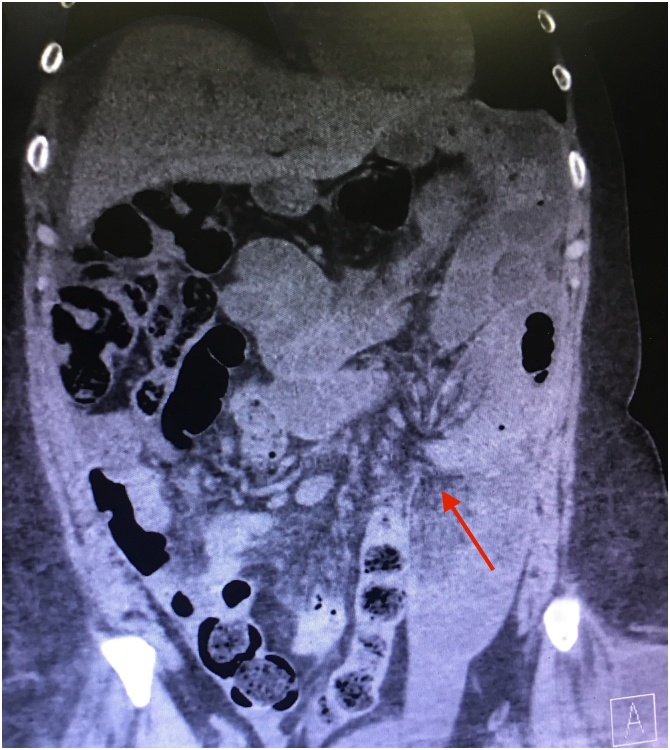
Computed tomography (CT) image of the retroperitoneal hernia. Red arrow shows where the small bowel is penetrating through the retroperitoneal space.
